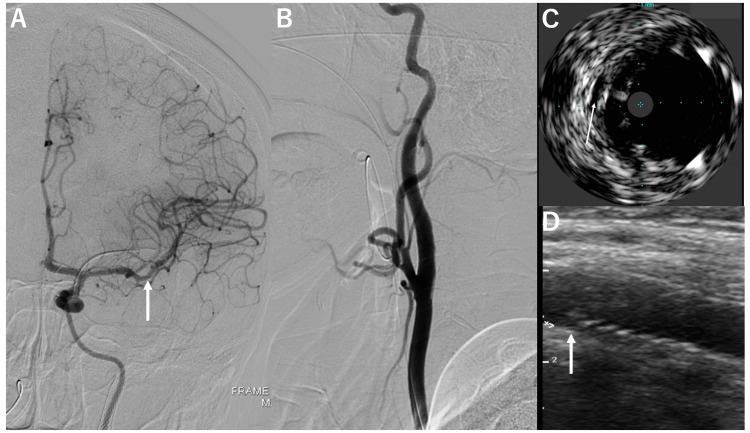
Final angiogram after treatment and postoperative imaging. A: Anterior-posterior view of the intracranial left internal carotid artery. The arrow indicates the reopened middle cerebral artery. B: Lateral view of the cervical carotid artery. C: Intravascular ultrasound image. D: Trans-surface carotid artery ultrasonogram. Arrows in C and D indicate the lumen outside the stent, and the true lumen is suspected.
